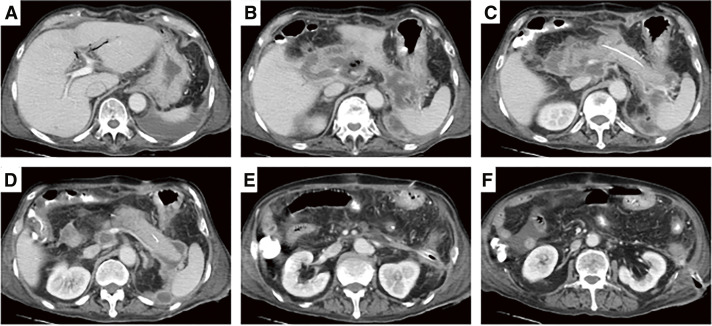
(A–F) On POD 82, contrast-enhanced CT revealed a residual abscess posterior to the stomach. POD, postoperative day; CT, computed tomography
